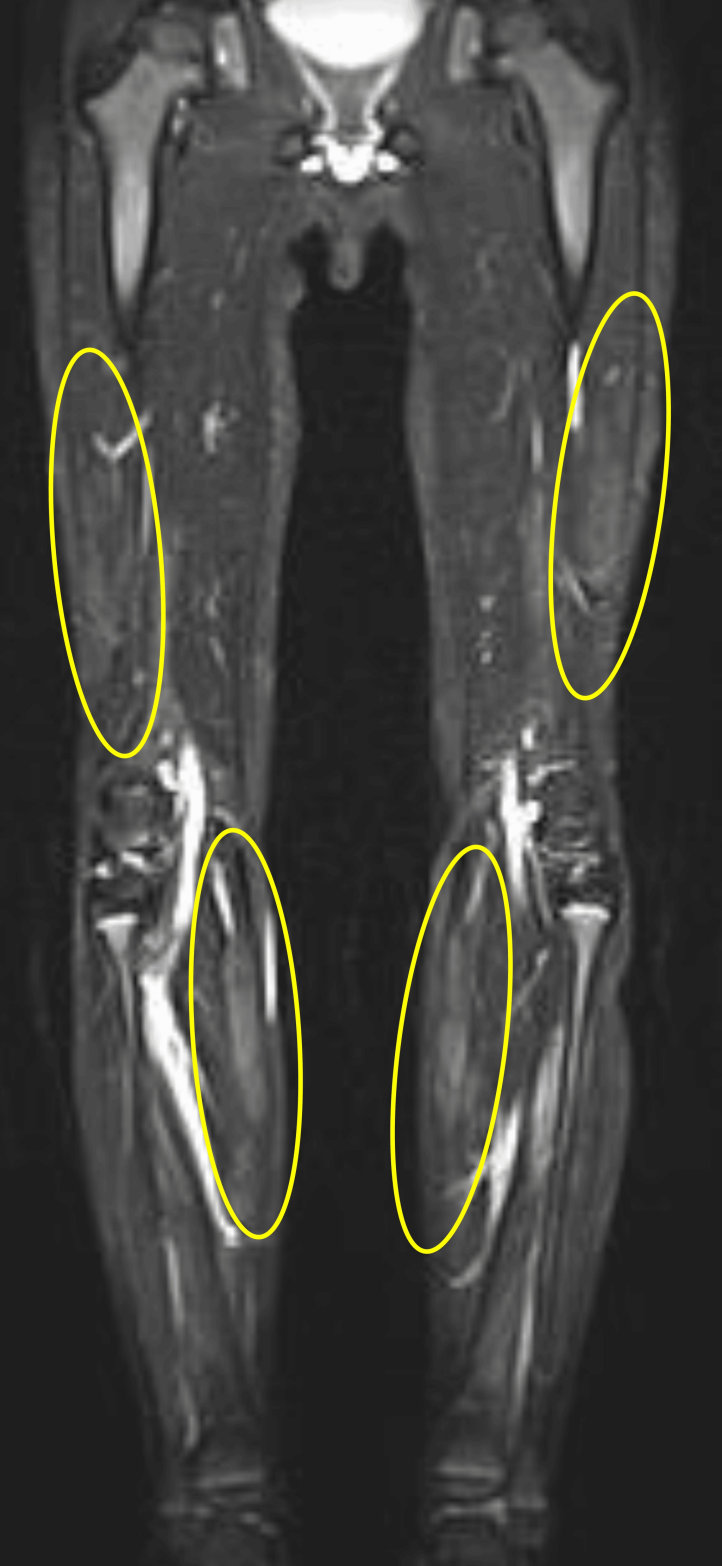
The coronal section of the MRI scan using STIR MRI: Magnetic resonance imaging; STIR: short tau inversion recovery
